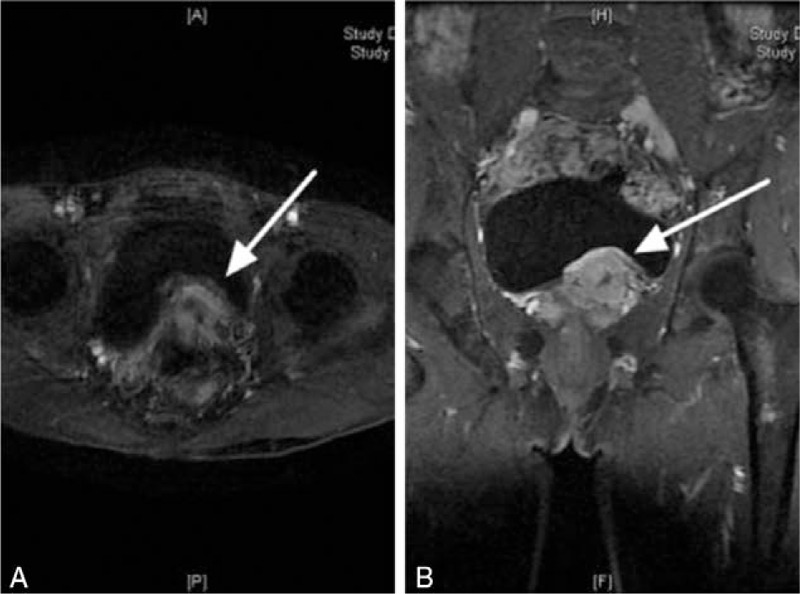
Magnetic resonance imaging (MRI): Transverse (A) and frontal (B) views indicate prostate tumor involving local central lobe (arrows).
